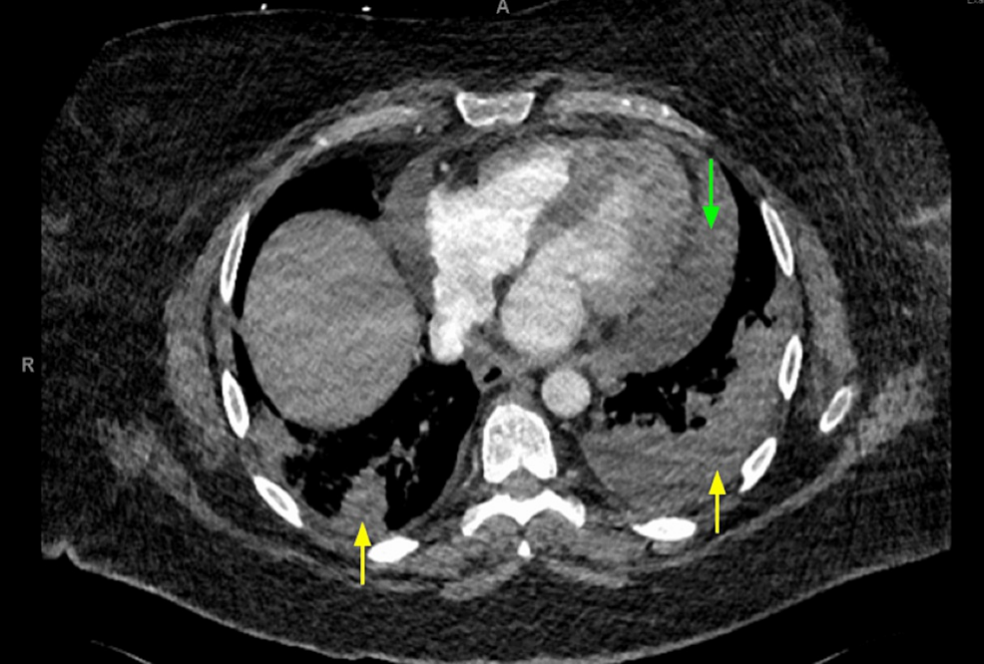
Pericardial effusion and pulmonary infarcts on CT chest Our patient with acute promyelocytic leukemia and prior pulmonary embolism presented with increasing dyspnea and fatigue. CT of the chest demonstrated pericardial effusion (green downward arrow) as well as evolving bilateral pulmonary infarcts (yellow upward arrows). She was managed with pericardiocentesis, ongoing therapeutic anticoagulation, and supportive treatment including supplemental oxygen.
